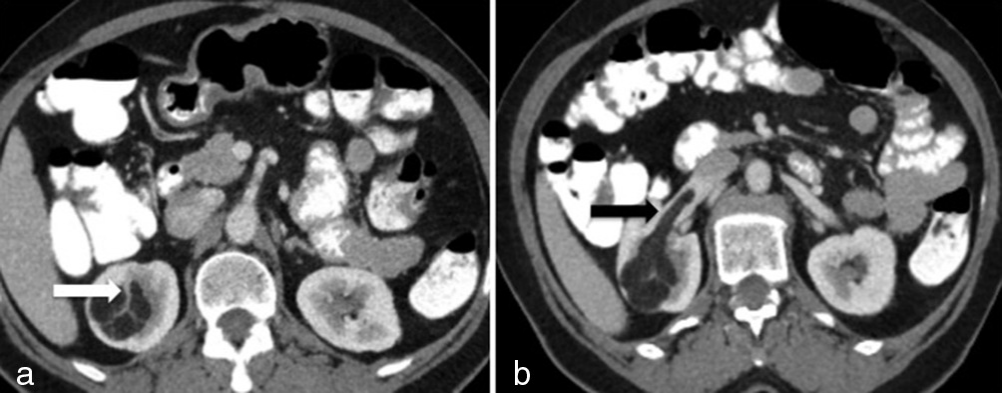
Contrast-enhanced axial CT images (a, b) show a right renal mass with fat attenuation causing a renal parenchymal defect. The mass contains visible tumour vessels extending into the renal parenchyma, a sign known as renal parenchymal vascular pedicle (white arrow; a). The fatty tumour thrombus filling the right renal vein (black arrow; b) is noteworthy.
